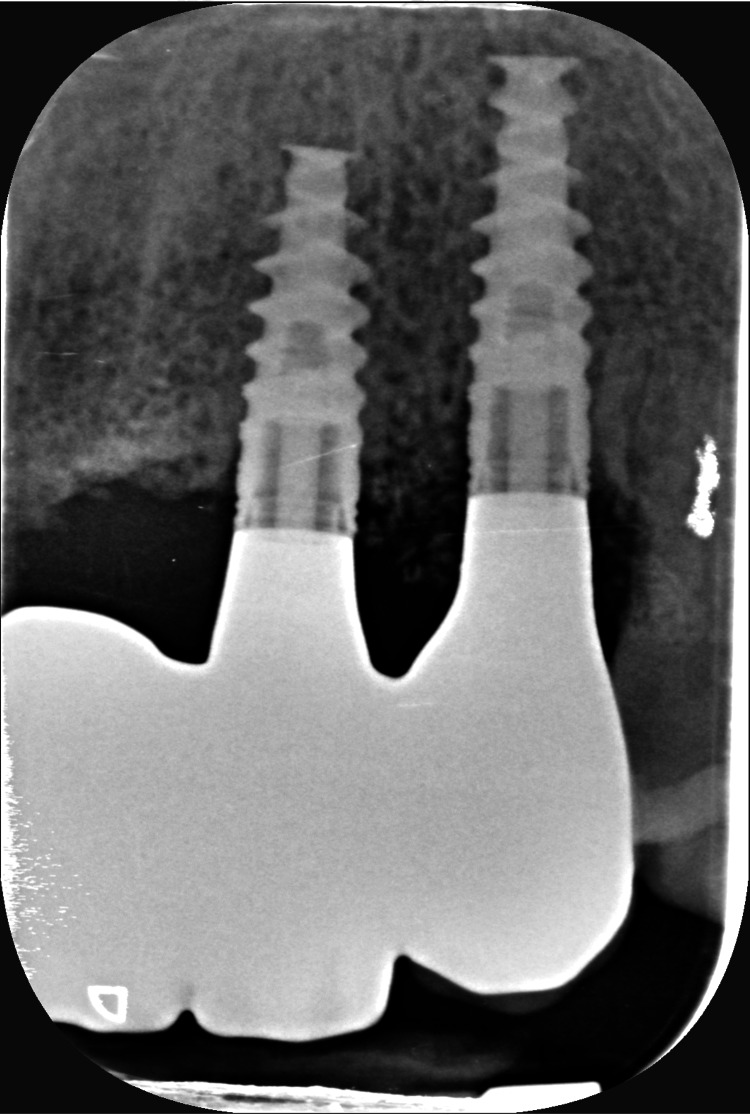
Intraoral radiograph of the framework screwed into implants #22 and #23
